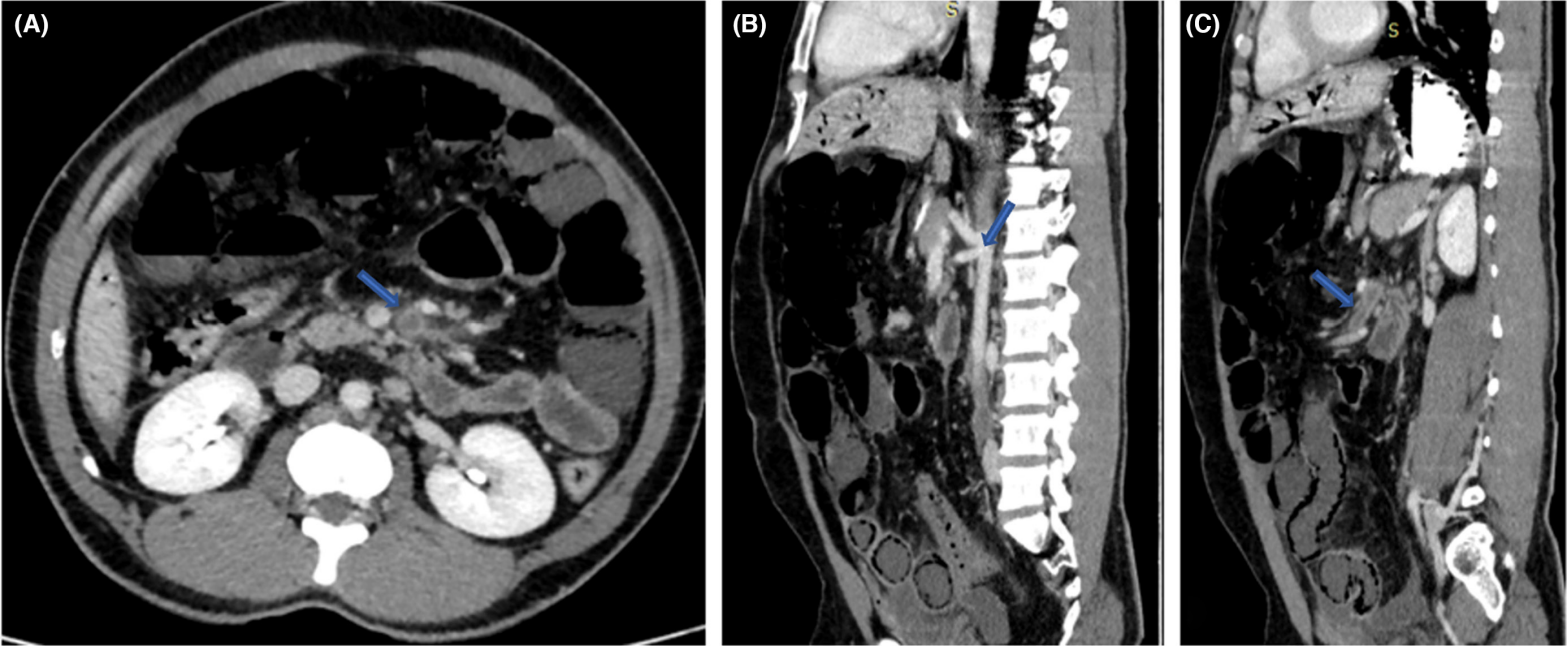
(A): Postcontrast axial CT image demonstrating filling defect in superior mesenteric artery (arrow). (B): Postcontrast sagittal CT image demonstrating celiac trunk and superior mesenteric artery with contrast opacification in their proximal aspect (arrow). (C): Postcontrast sagittal CT image demonstrating nonenhancing filling defect in superior mesenteric artery starting from approximately 2 cm distal to its origin (arrow). Figure 2 (B) and (C) also demonstrates air attenuating areas within the bowel wall (pneumatosis intestinalis) and minimal free fluid in pelvic peritoneal cavity.
